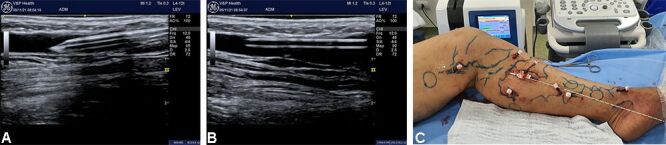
Images showing the multiple punctures made for treatment of tributaries and the great saphenous vein. (A and B) Ultrasound-guided placement of No. 16 IV catheter; (C) Appearance of the multiple punctures.5 - Several different segments of the tributary veins, saphenous or otherwise, can be punctured at a distance of at least 7 to 10 mm from the skin (measured with ultrasound after perivenous infiltration of 0.9% saline);6 - The patient is placed in the Trendelenburg position in order to provoke venous emptying;7 - The 600 micra bare tip or radial laser fiber is inserted, adjusting the position of the fiber tip to 2 cm from the SFJ or saphenopopliteal junction (SPJ) (Figure 2);
